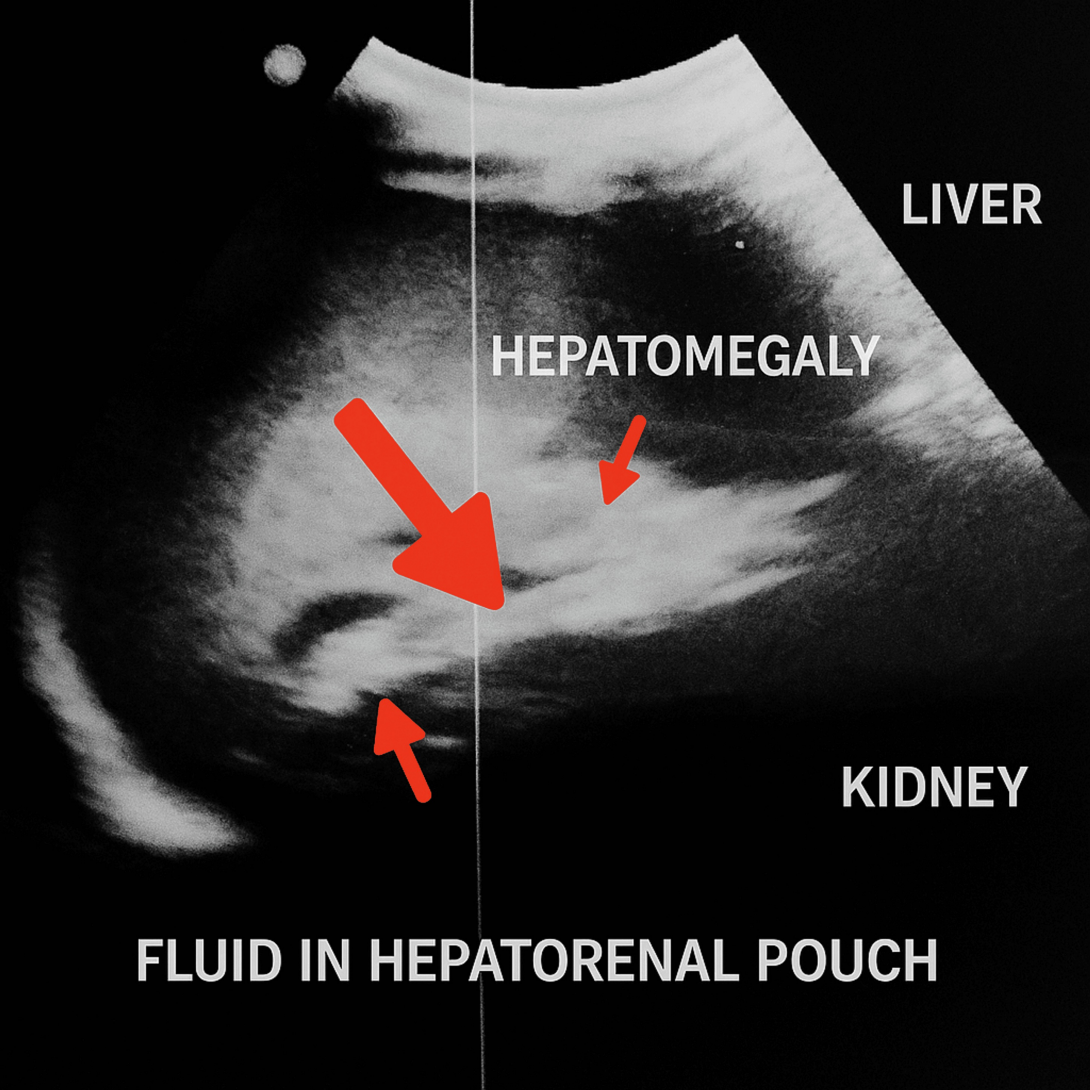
Ultrasound scan of the abdomen shows mild hepatomegaly along with a small amount of free fluid.
